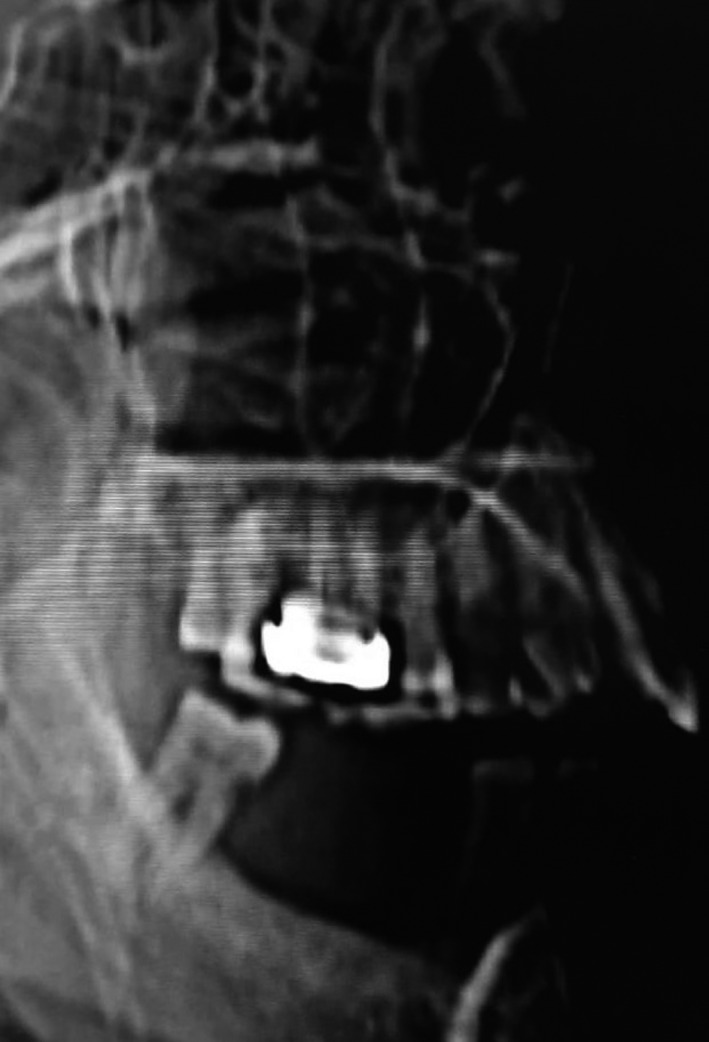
CT view depicting dentition and sinus floor
